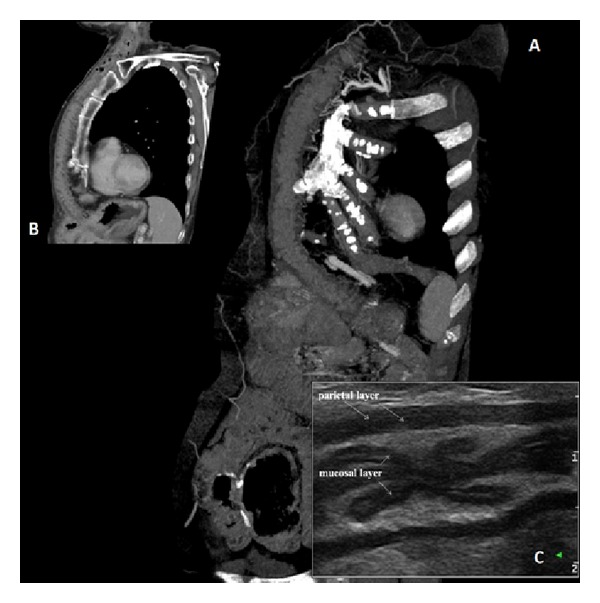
((a)-(b)) Sagittal view CT images show the normal esophageal bypass position without signs of dilatation or ischemic suffering. (c) US image allows an optimal evaluation of mucosal and parietal layers in the whole length of the organ.
